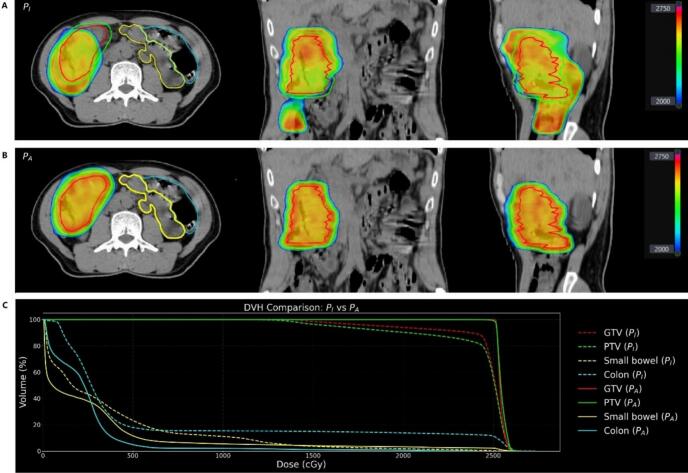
Dose comparison between the initial reference plan and the adapted plan on the daily CT image. (A) Initial reference plan (PI) recalculated on the daily CT. (B) Adapted plan (PA) generated after re-contouring and re-optimization. (C) Dose–volume histogram (DVH) comparing the two plans, with solid lines representing the adapted plan and dashed lines representing the initial plan. Structures are color-coded as follows: gross tumor volume (GTV, red), planning target volume (PTV, green), small bowel (yellow), and uninvolved colon (blue).
